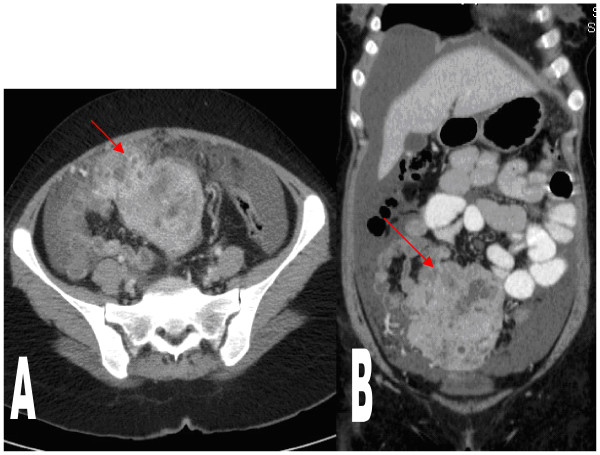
(A, B) Computed tomography (CT) axial and coronal cuts showing the pelvic/abdominal heterogeneous mass with areas of necrosis in addition to ascites.
